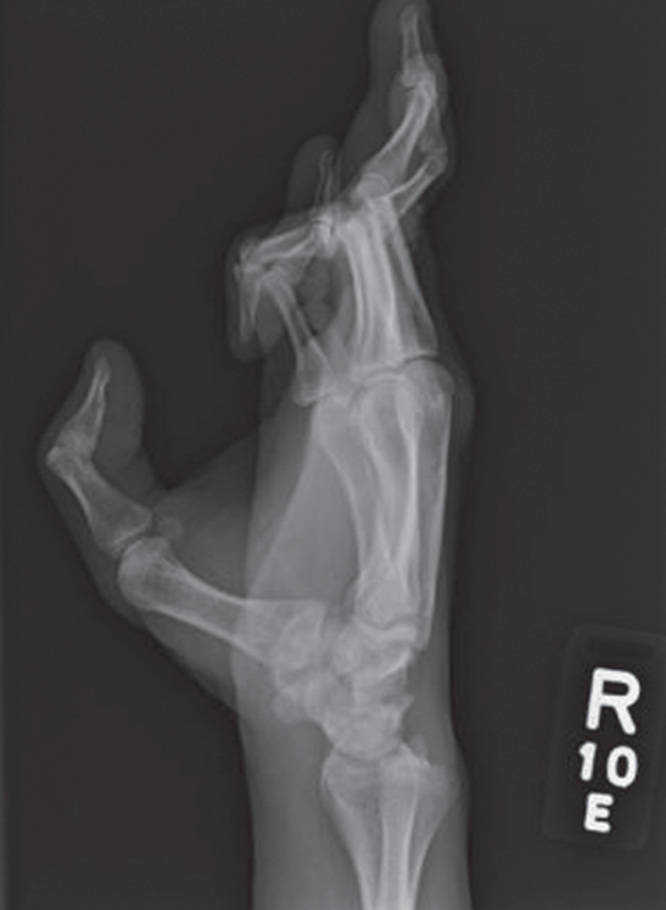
Lateral preoperative x-rays. The patient demonstrates Z deformity and dislocation of first metacarpal. No arthritic changes are evident over the joint surfaces
